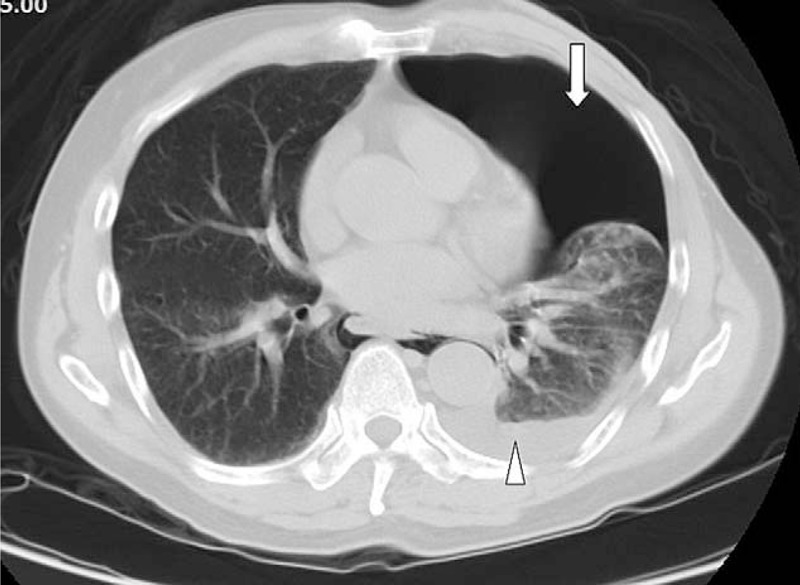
Emergent contrast thoracic computerized tomography (CT) demonstrating massive pneumothorax on the left side (arrow) with collapsed lung and mild pleural effusion (arrowhead).
